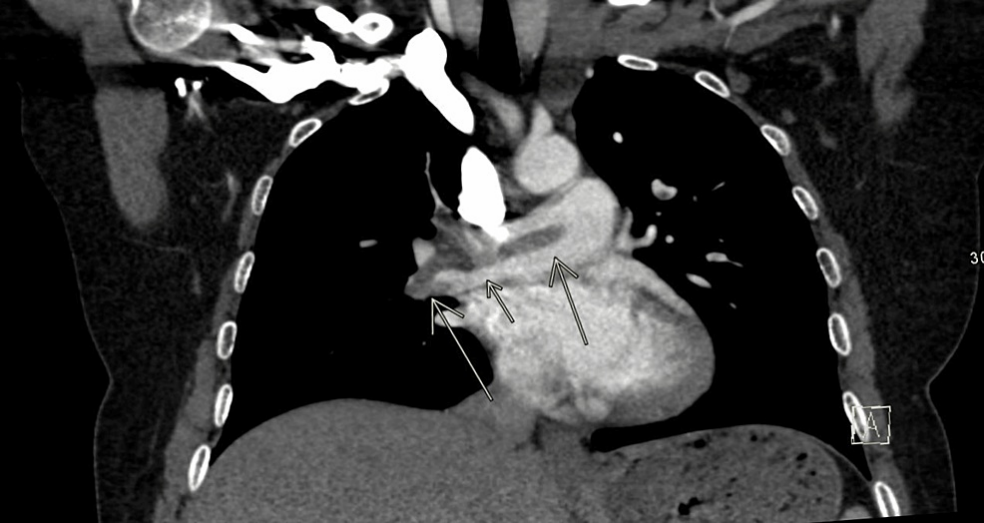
Coronal pulmonary CT angiogram demonstrating the longest in-plane view of the thrombus seen across the pulmonary arteries.
